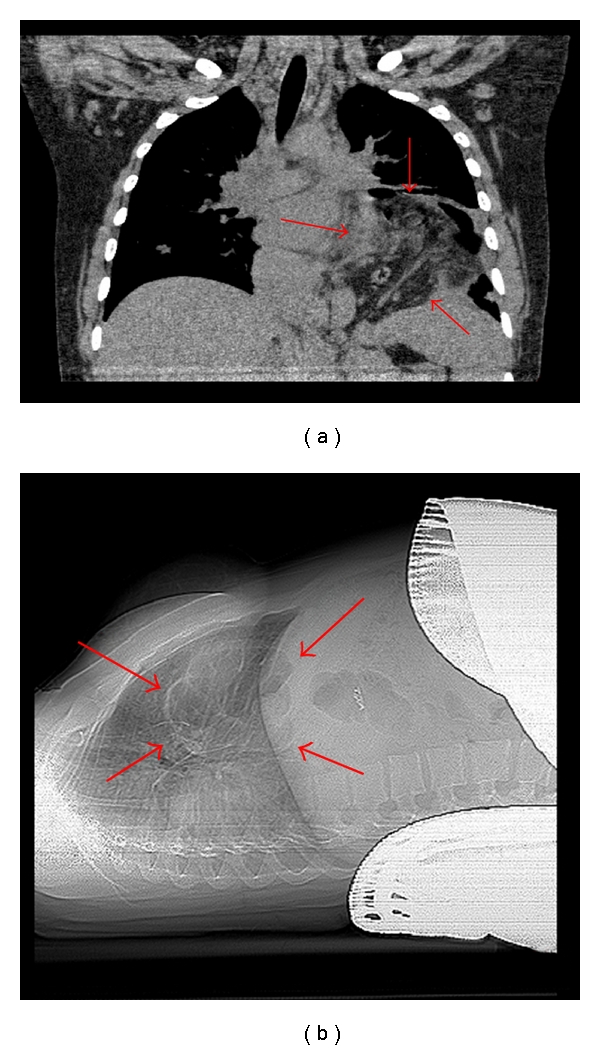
(a) CT of the chest showing the large diaphragmatic hernia (shown by the arrows). The hernia is compressing the left lung and has caused some slight deviation of the trachea to the contralateral side of the chest. (b) Lateral view of diaphragmatic hernia (outlined by arrows) with patient laying supine.
